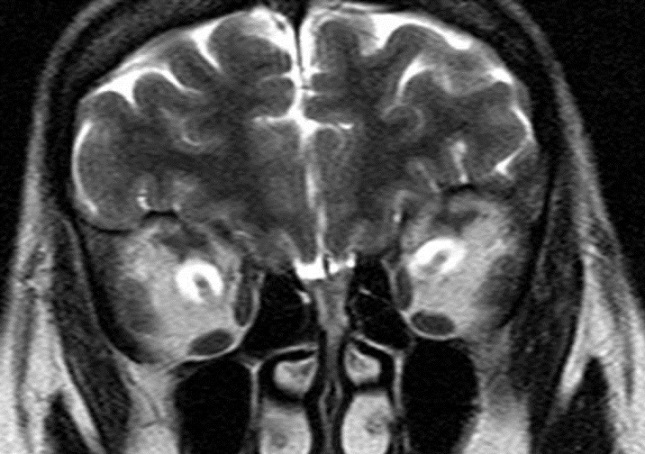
Orbital MRI; Coronal view of T2-weighted MRI Hyperintense cerebrospinal fluid-intensity dilated sheath surrounding normal optic nerves (bull's eye)
